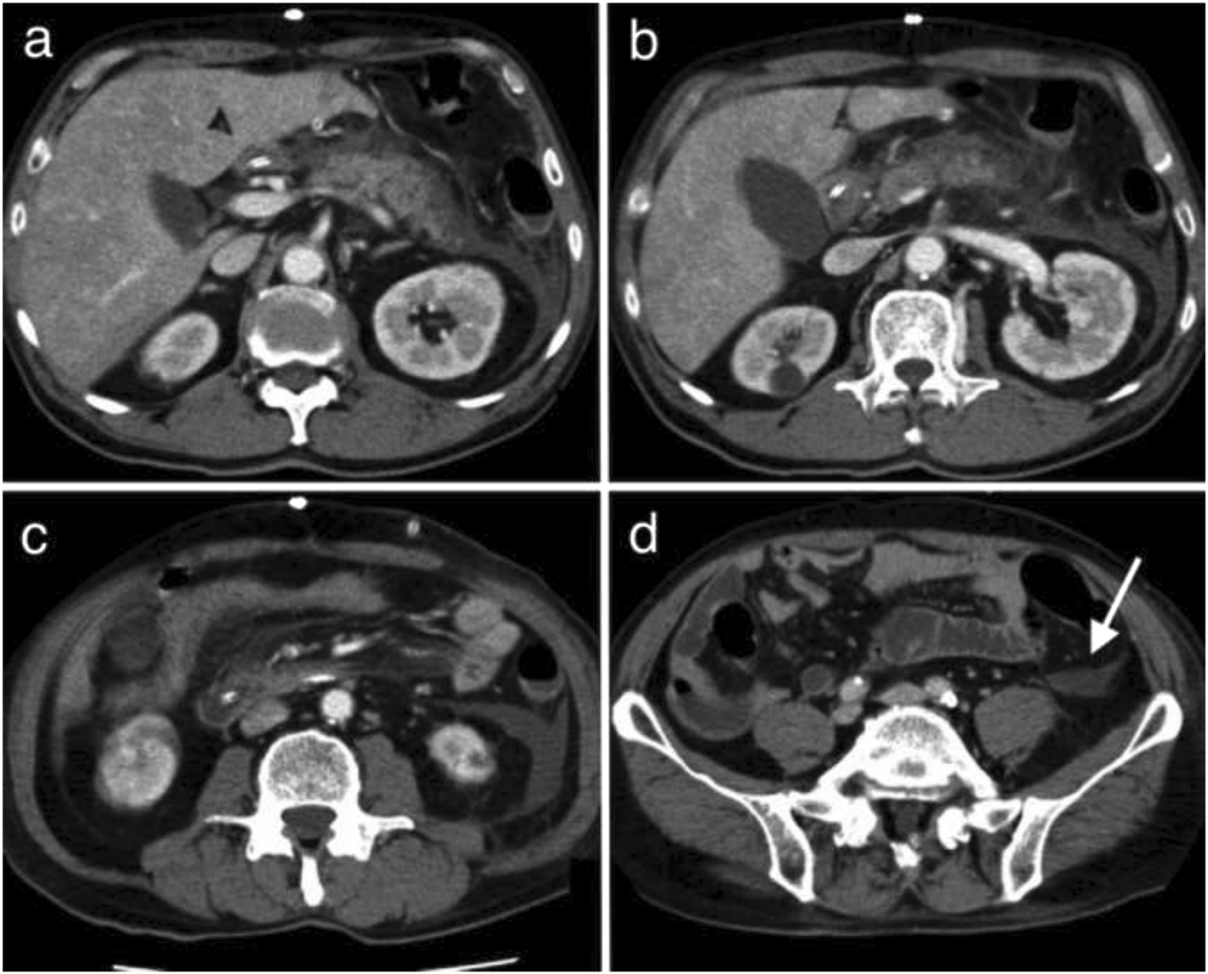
Abdominal contrast computed tomography findings. Increased density of adipose tissue surrounding the pancreas and some areas without contrast enhancement in the pancreatic body was observed (a-c). Furthermore, inflammation extended from the inferior pole of the left kidney to the pelvic region (d). On the basis of the findings on the computed tomography scans, the patient was diagnosed with severe acute grade 3 pancreatitis (Japanese Guidelines for diagnosis of acute pancreatitis 2010, third version).
